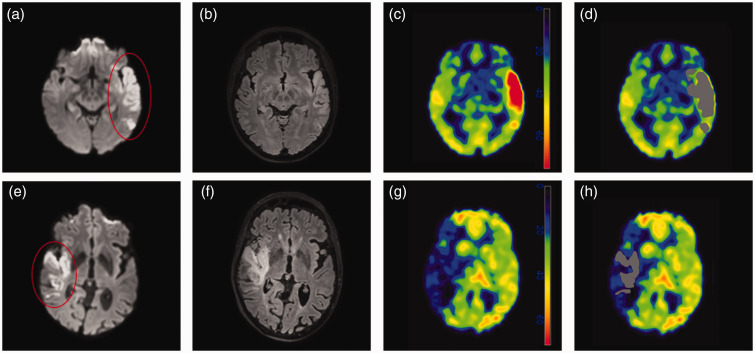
Exemplary patient data. Diffusion-weighted images (DWI, a, e), fluid-attenuated inversion recovery images (FLAIR, b, f), and cerebral blood flow (CBF, c, d, g, h) maps are shown for two exemplary patients. Additionally, DWI-derived masks (in grey) are overlayed onto CBF maps (d, h), indicating the volume of interest in which the CBF map was evaluated for further analyses. The color-coded bands for units in c and g are ml_Blood_/100 g_Tissue_/min. *Patient 1 (a–d):* A 61-year-old woman with a history of sudden onset facial paresis and dysarthria (baseline National Institutes of Health Stroke Scale [NIHSS]: 6). Thrombectomy was performed successfully with an expanded Treatment in Cerebral Infarction (eTICI) 3 result. (a) DWI demonstrates the infarct focus at the left insula and temporal lobe (red circle). (b) On the FLAIR image, subtle parenchymal hyperintensity (swelling) at the left temporal cortex is seen. (c) Five days after thrombectomy, perfusion increase appears at the corresponding left middle cerebral artery (MCA) territory using pseudo-continuous arterial spin labeling (pCASL). *Patient 2 (e–h):* A 77-year-old woman with a history of sudden onset left brachiocephalic hemiparesis, dysarthria, and left-sided neglect (baseline NIHSS: 7). Thrombectomy was performed successfully with an eTICI 3 result. (e) DWI demonstrates the infarct focus at the right insula and temporal lobe (red circle). (f) On the FLAIR image, parenchymal hyperintensity is shown in the corresponding area. (g) Five days after thrombectomy, pCASL shows hypoperfusion at the right MCA territory. This is an exemplary case of the no-reflow phenomenon.
